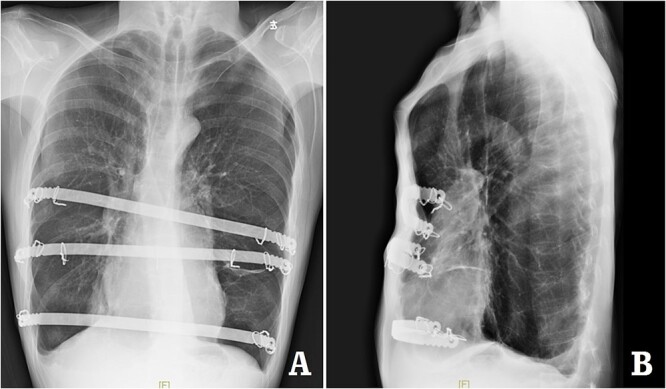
X-ray examination after operation. (A) Posteroanterior radiograph; and (B) lateral radiograph.
